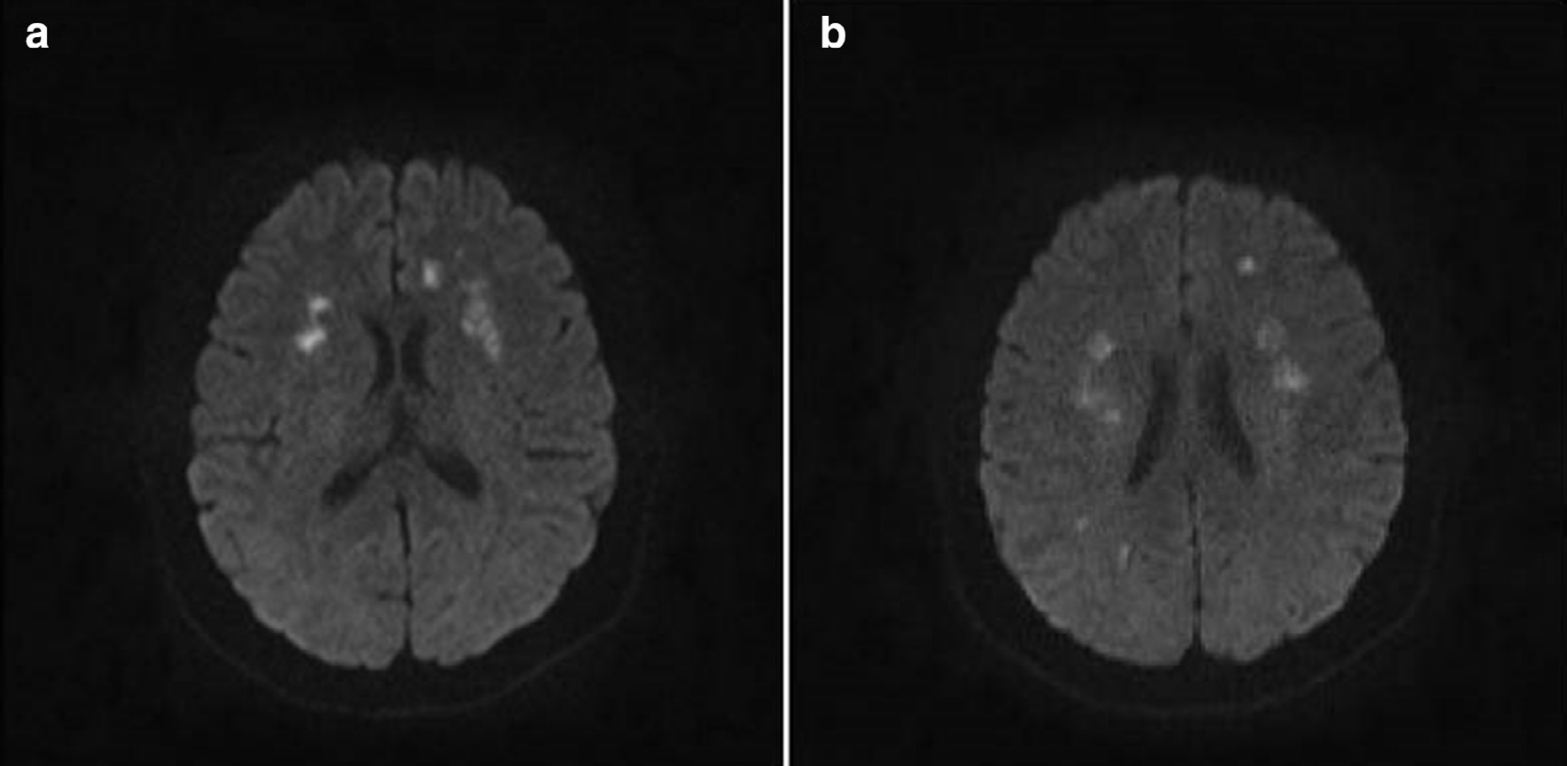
Axial DWI MR images A and B shows acute infarcts in bilateral corona radiata.
